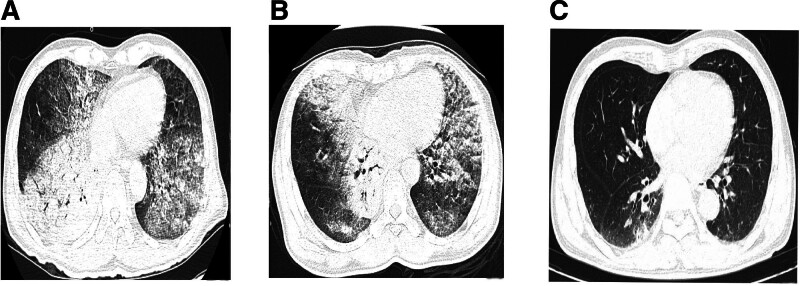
Chest CT: (A) On admission in the supine position showed diffuse ground-glass opacities in both lungs, thickened interlobular septa, and consolidation in the right lower lobe; (B) in the prone position after 2 days of APP; (C) in supine position 1 month after targeted therapy. APP = awake prone position, CT = computed tomography.
